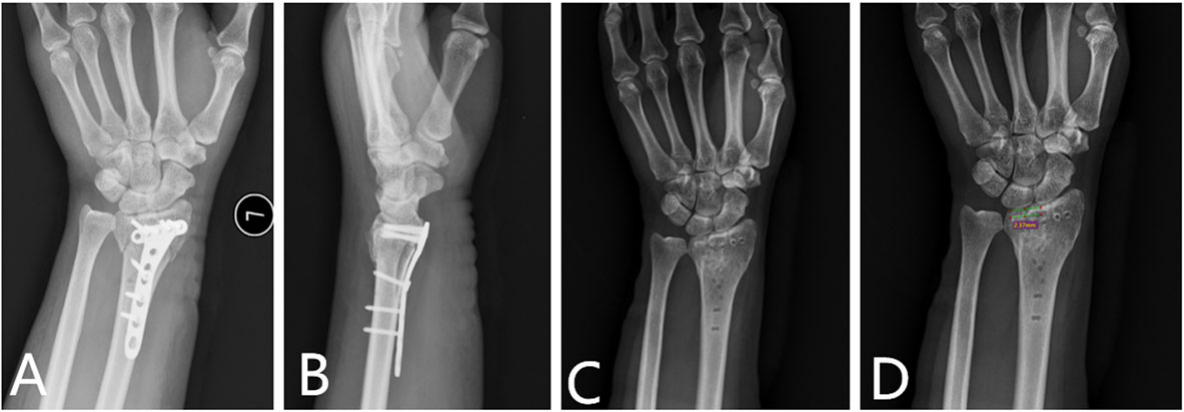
The immediate postoperative X-rays (a and b) showed good reduction and VLP fixation in a 44-year woman who had left wrist fracture (AO type B1). The X-rays (c and d) after removal of VLP at postoperative 14 months showed collapsed of the lunate fossa (2.37 mm)
